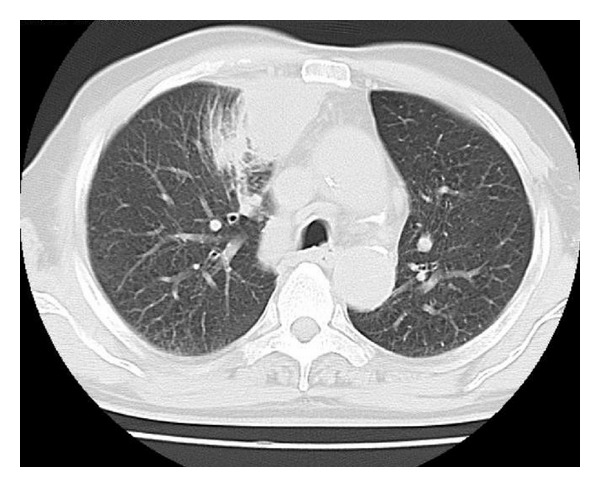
Spiral CT of the thorax showing an irregular mass lesion in the anterior and superior mediastinum.
